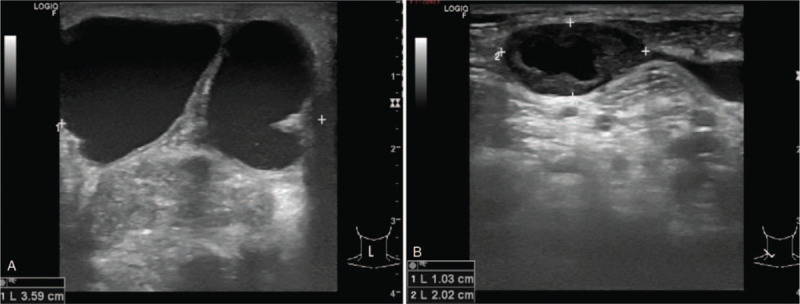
(A) Neck ultrasonography at the last presentation showed heterogeneous echogenicity of solid lesion with multiple internal calcifications at right thyroid bed (size 2.1 × 1.6 × 2.8 cm with adjacent complex multilocular cystic lesion with septations and (B) enlarged right cervical lymph node with absent fat hilum.
